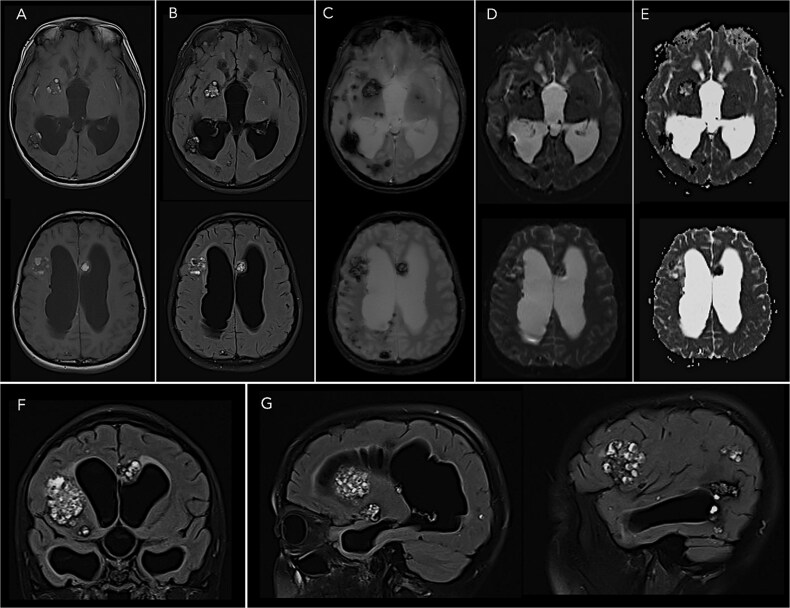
Axial T1*-weighted (A), T2*-weighted fluid-attenuated inversion recovery (B), gradient-recalled echo (C), diffusion-weighted (D), and apparent diffusion coefficient (E) magnetic resonance images demonstrating multiple lobulated complex masses containing blood products of various ages with associated hemosiderin rims consistent with cerebral cavernous malformations in the bilateral cerebral hemispheres, right thalamus, and bilateral capsuloganglionic regions. There is marked dilatation of the ventricular system. Coronal (F) and sagittal (G) T2*-weighted fluid-attenuated inversion recovery magnetic resonance images are also shown.
